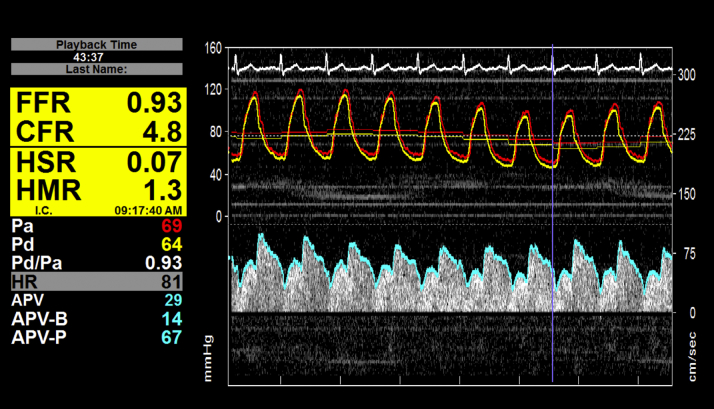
Figure Demonstrating an Example of Invasive Pressure and Doppler Flow Measurements APV = average peak flow velocity; APV-B = average peak flow velocity at baseline; APV-P = average peak flow velocity at peak hyperemia. CFR = coronary flow reserve; FFR = fractional flow reserve; HMR = hyperemic microvascular resistance; HR = heart rate; HSR = hyperemic stenosis resistance; Pa = aortic pressure; Pd = distal coronary pressure.
